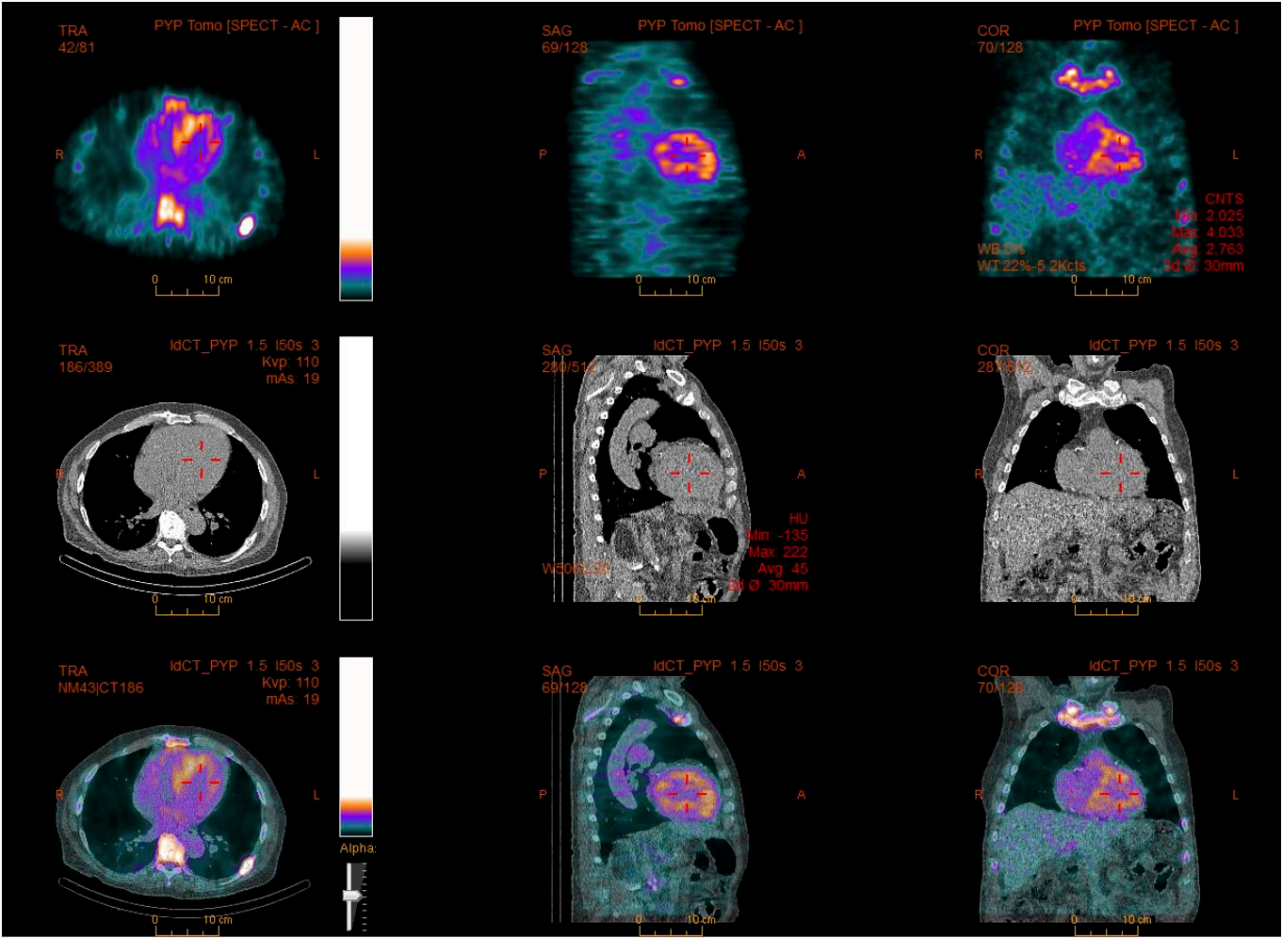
Family 1, Patient 1: SPECT/CT showing a myocardial uptake of the radiotracer.
